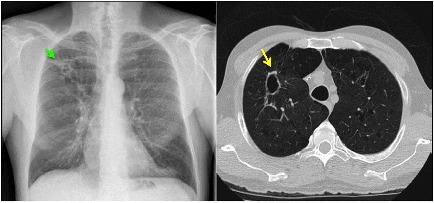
Left: chest radiograph demonstrating a right upper lobe cavitary lesion on a background of emphysema (green arrow). Right: axial CT image demonstrating the same cavitary lesion in the right lung (yellow arrow). Reprinted with permission of the American Thoracic Society. Copyright© 2018 American Thoracic Society. Fennelly et al. (2016) is an official journal of the American Thoracic Society.
